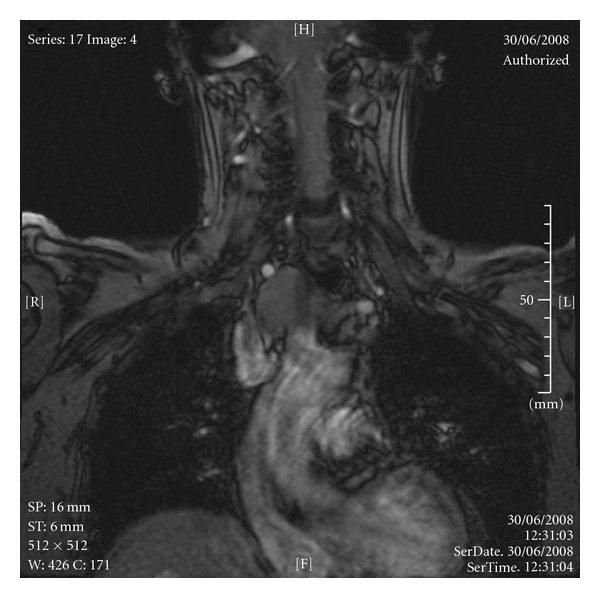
Anteroposterior magnetic resonance image.
